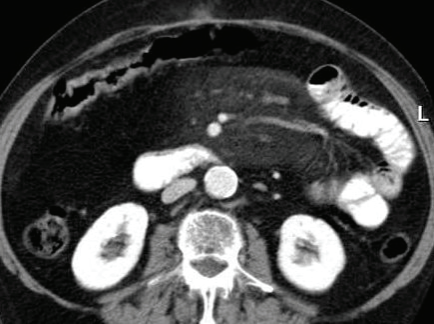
Axial contrast enhanced CT image of the mid abdomen shows a heterogeneous fibrofatty mass within the root of the mesentery containing a focus of calcification.
